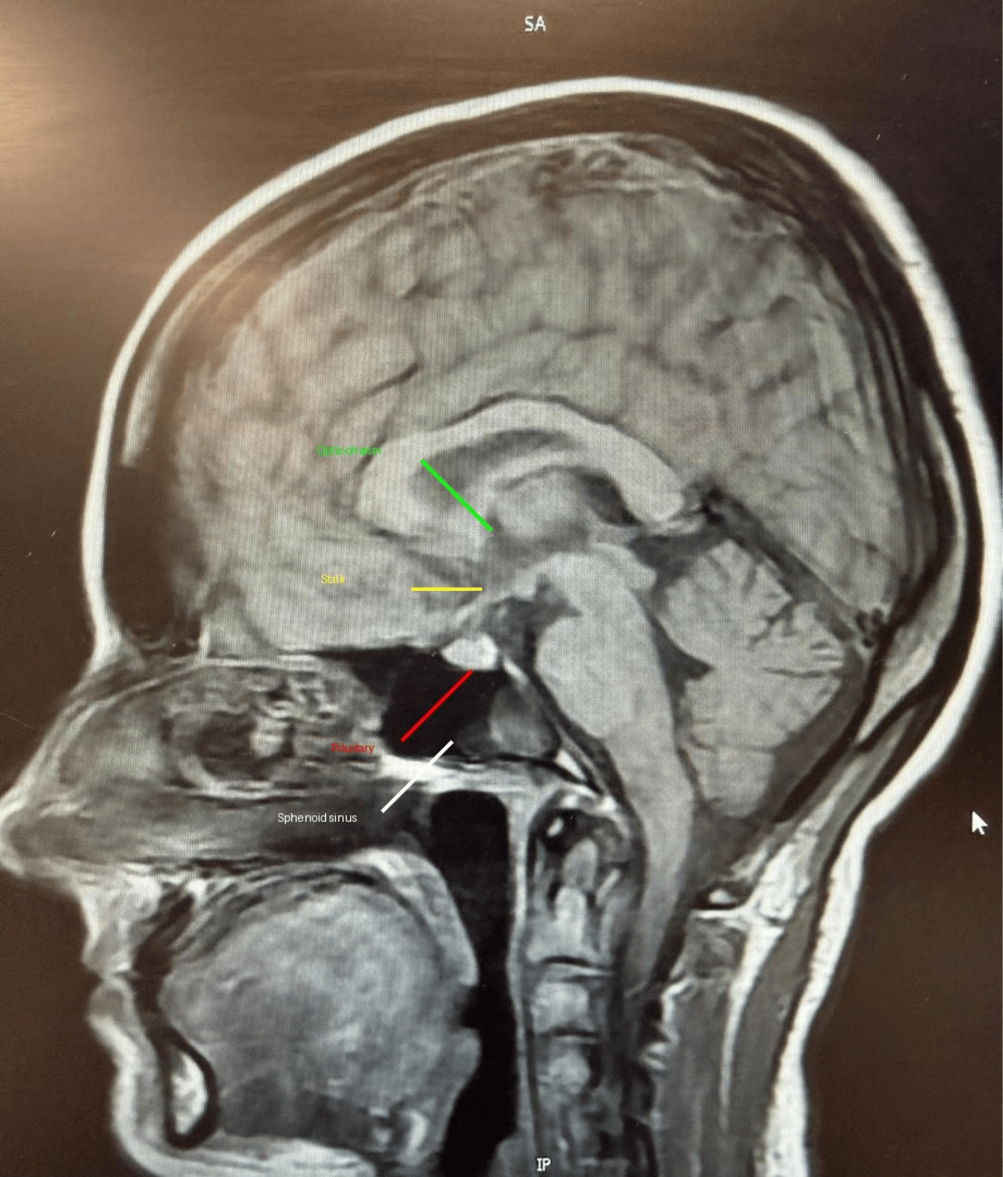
Sagittal MRI brain (midline view) Sagittal T1-weighted magnetic resonance imaging (MRI) of the brain demonstrates preserved midline anatomy. The pituitary gland is normal in size and contour, with no evidence of haemorrhage or mass lesion. There is no suprasellar extension or compression of the optic chiasm.
